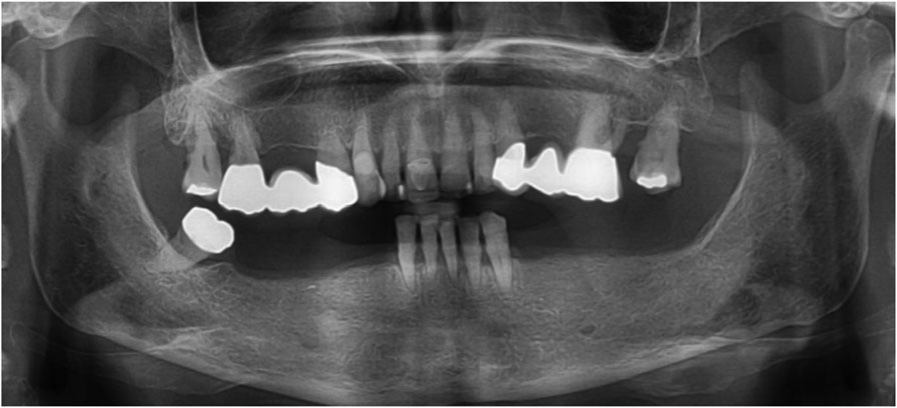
Panoramic radiograph of the initial dental status
